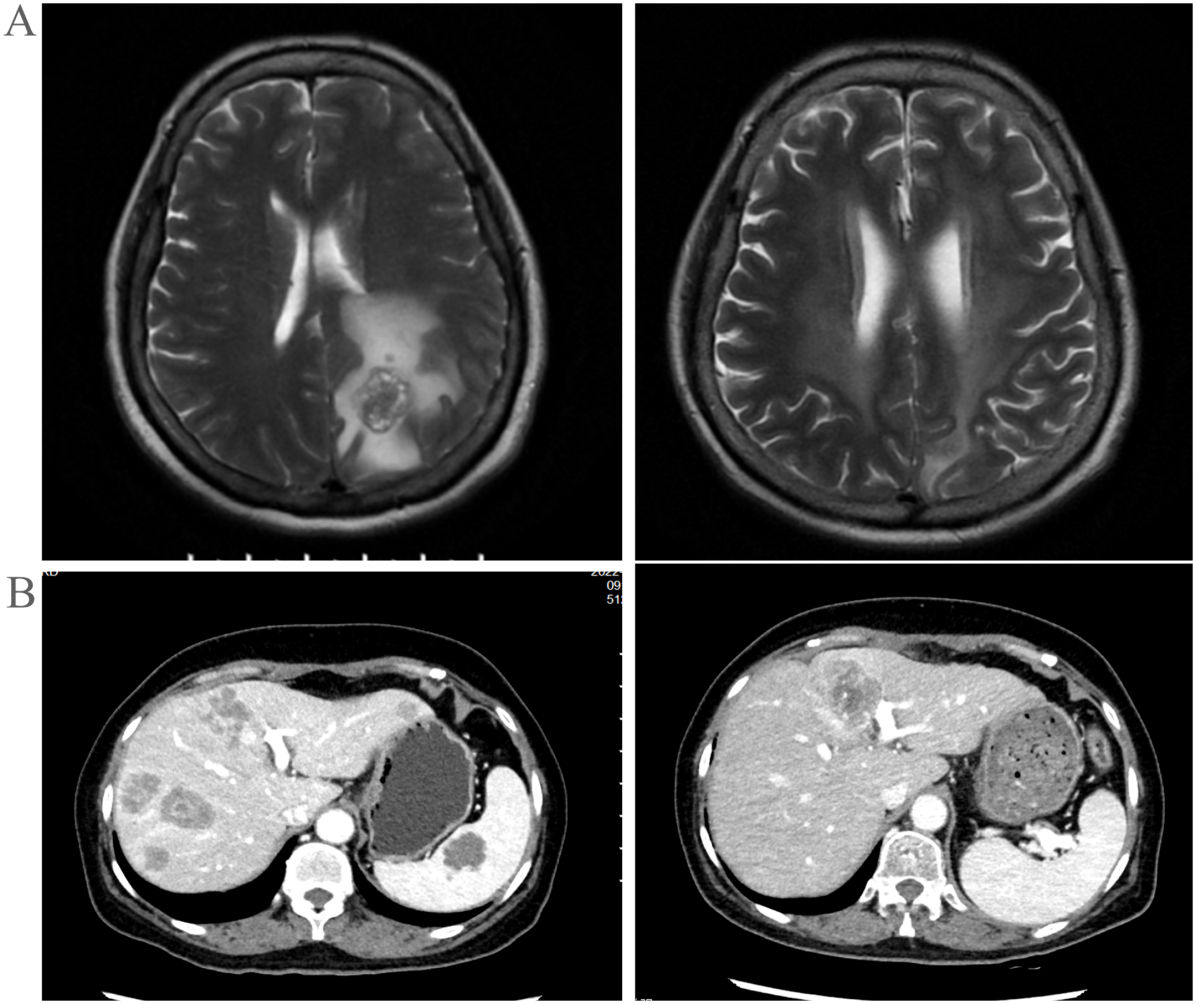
(A) Extra-pulmonary organs: head, MRI images before and after treatment. (B) Extra-pulmonary organs: liver, CT images at key points during the treatment process.
